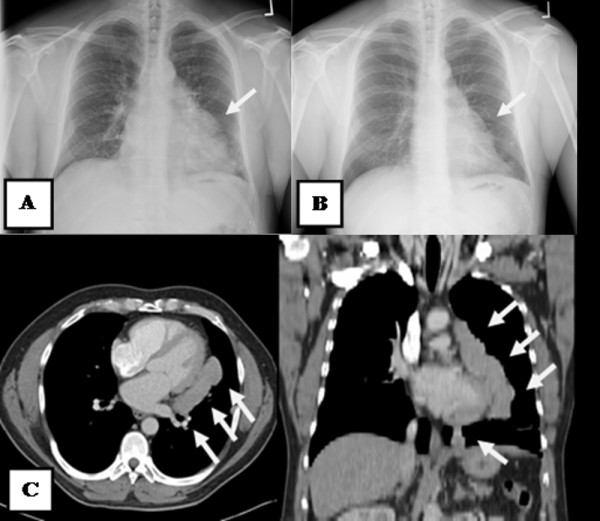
Chest X-rays (A) at presentation; (B) 1 year previously; (C) Computed tomography demonstrated a homogenously enhanced mass (arrows).
